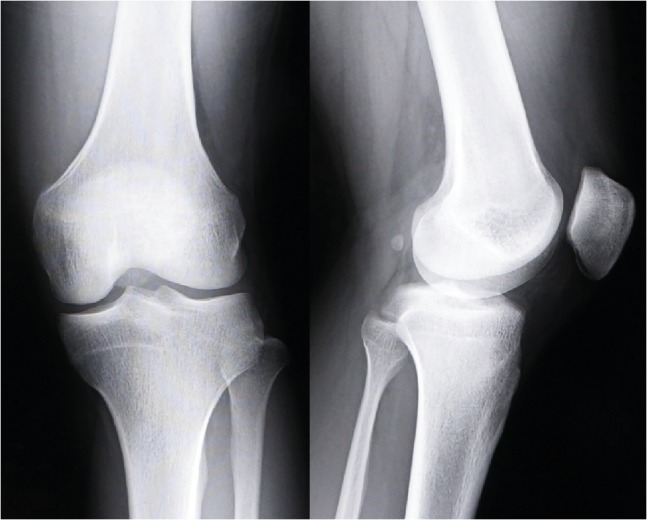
Plain radiograph obtained preoperatively showing no abnormalities.
